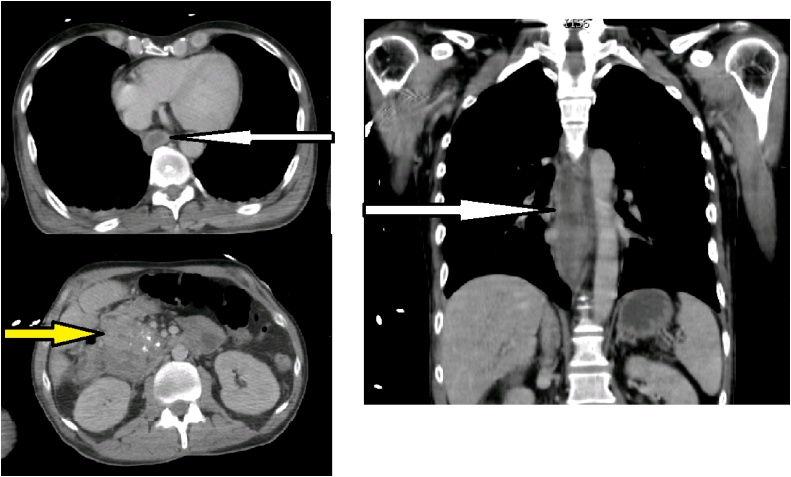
CT-scan with stagnant fluid in esophagus (white arrow) and thickening of the duodenal walls (yellow arrow).
